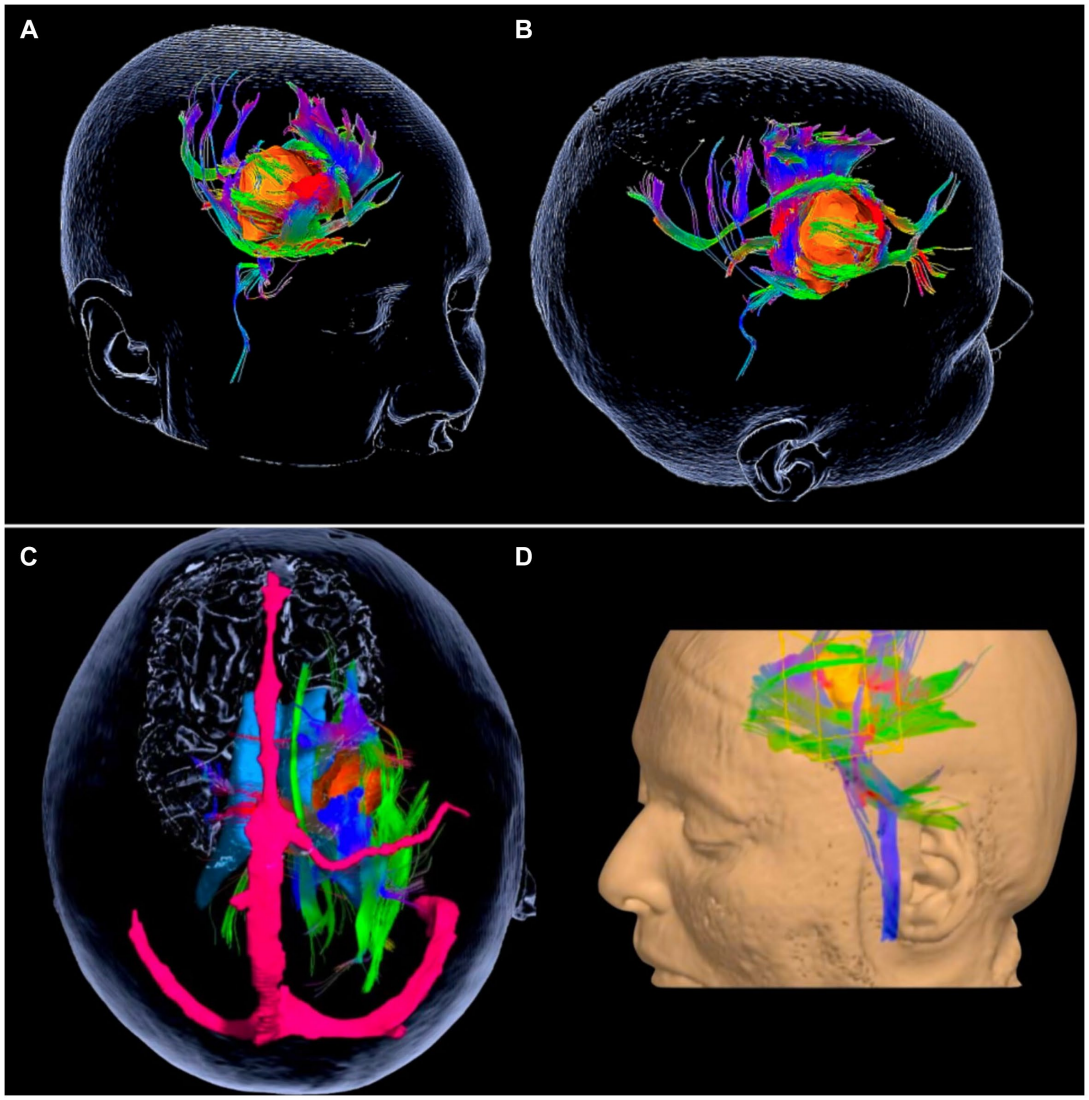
The usefulness of tractography in cavernomas. (A,B) Cavernoma of the anterior limb of the internal capsule. 3D reconstruction of the MRI tractography showing the relationship of the cavernosum with the nuclei of the base and adjacent white encephalic fibers. Cavernoma in orange, association fibers (green) displaced medially and laterally, projection fibers (blue and violet) displaced predominantly posteriorly and anteriorly. (C,D) Cavernosa of the posterior limb of the internal capsule. Cavernoma (orange). Projection fibers (blue), long association fibers, or fascicles (green). Superior sagittal sinus and vein of Trolard (in pink). (B) Reconstruction showing the lesion in the left frontal lobe. Note the displacement of the internal capsule projection fibers predominantly anteriorly and in smaller volumes posteriorly.
